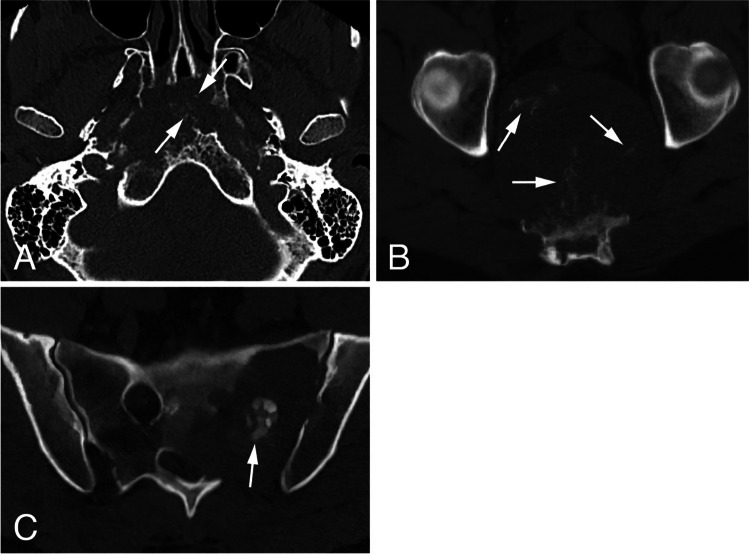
Examples of different calcification types: A- Axial view CT scan of a skull base chondrosarcoma showing *rings-and-arcs/popcorn*-like calcification (arrows). B- Axial view CT scan of sacral chordoma showing linear bone remnants (*amorphous*-like calcification) consistent with pre-existing bone (arrows). C- Axial view CT scan of sacral osteosarcoma presenting with *dense sclerotic*-like (osteoid) calcification (arrow)
